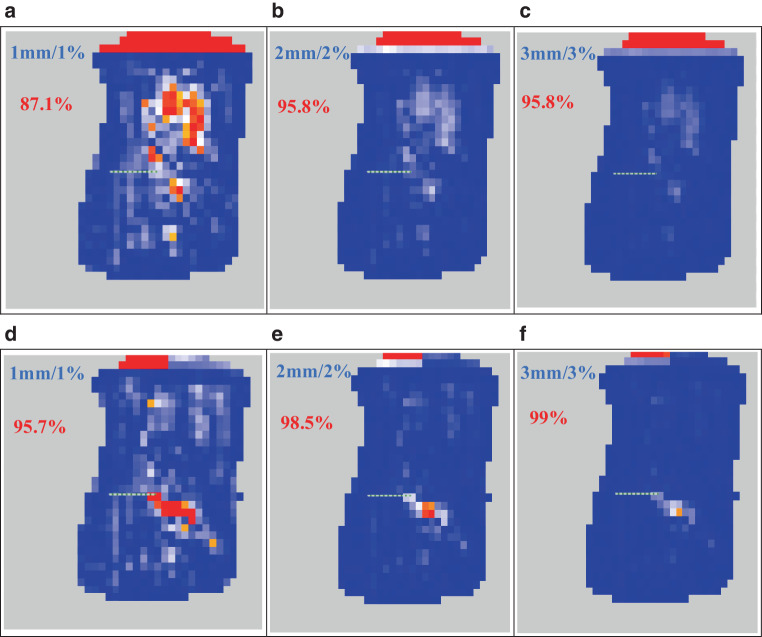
The gamma analysis index distribution calculated on original cone-beam CT (CBCT; a, b, c) and generated synthetic CT (sCT; d, e, f) images, with dose on the deformed CT image as reference using entire intensity-modulated radiation therapy fields for one test patient using three criteria
